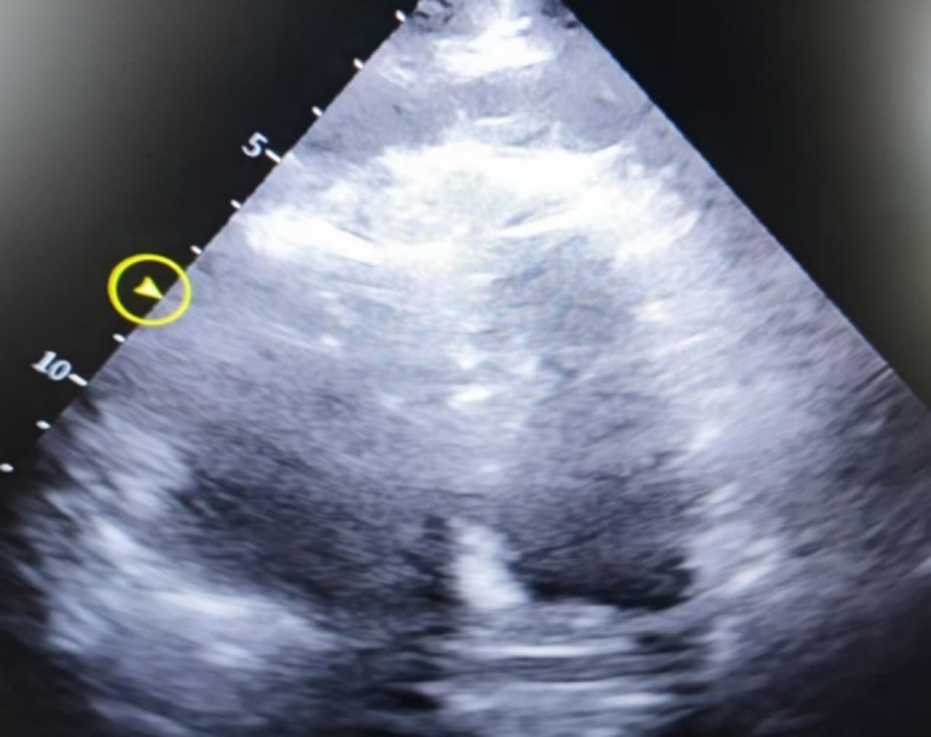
Apical four-chamber heart image, showing decreased left ventricular systolic function, severe dilatation of the right atrium and right ventricle, with the right ventricle measuring approximately 6.51 × 4.58 cm.
